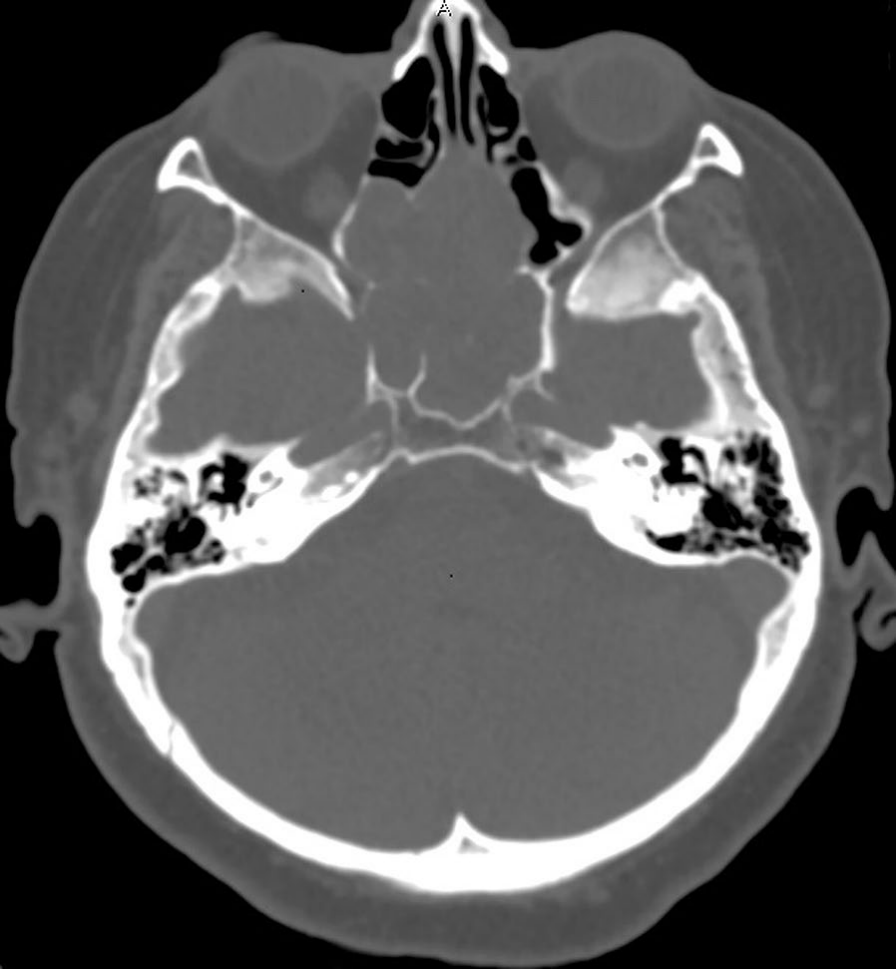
Computed tomography of paranasal sinuses. Axial projection. Hipodensive masses in the posterior ethmoid and sphenoid sinuses with expansion to nasal cavities. Partially pneumatised ethmoid cells are visible
